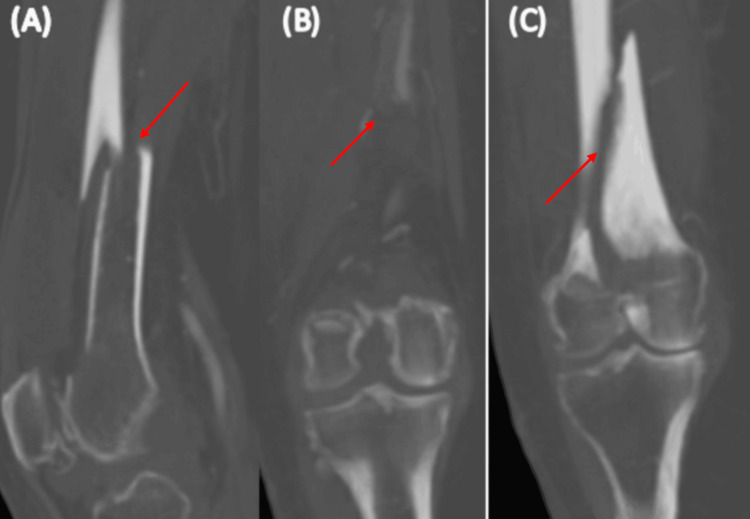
A computed tomography (CT) scan of the right femur Panel (A) is a sagittal view, while panels (B) and (C) are coronal views of the distal femur. There is a displaced longitudinal fracture line in the distal femur diaphysis extending into the joint surface, as shown by the arrow in panels (B) and (C). The fracture passes through the intercondylar notch, with the largest medial fragment displaced posteriorly, as shown by the arrow in Panel (A). The lateral femoral epimetaphysis shows an impacted fracture. This suggests a complex fracture involving both displacement and impaction, affecting the joint surface.
